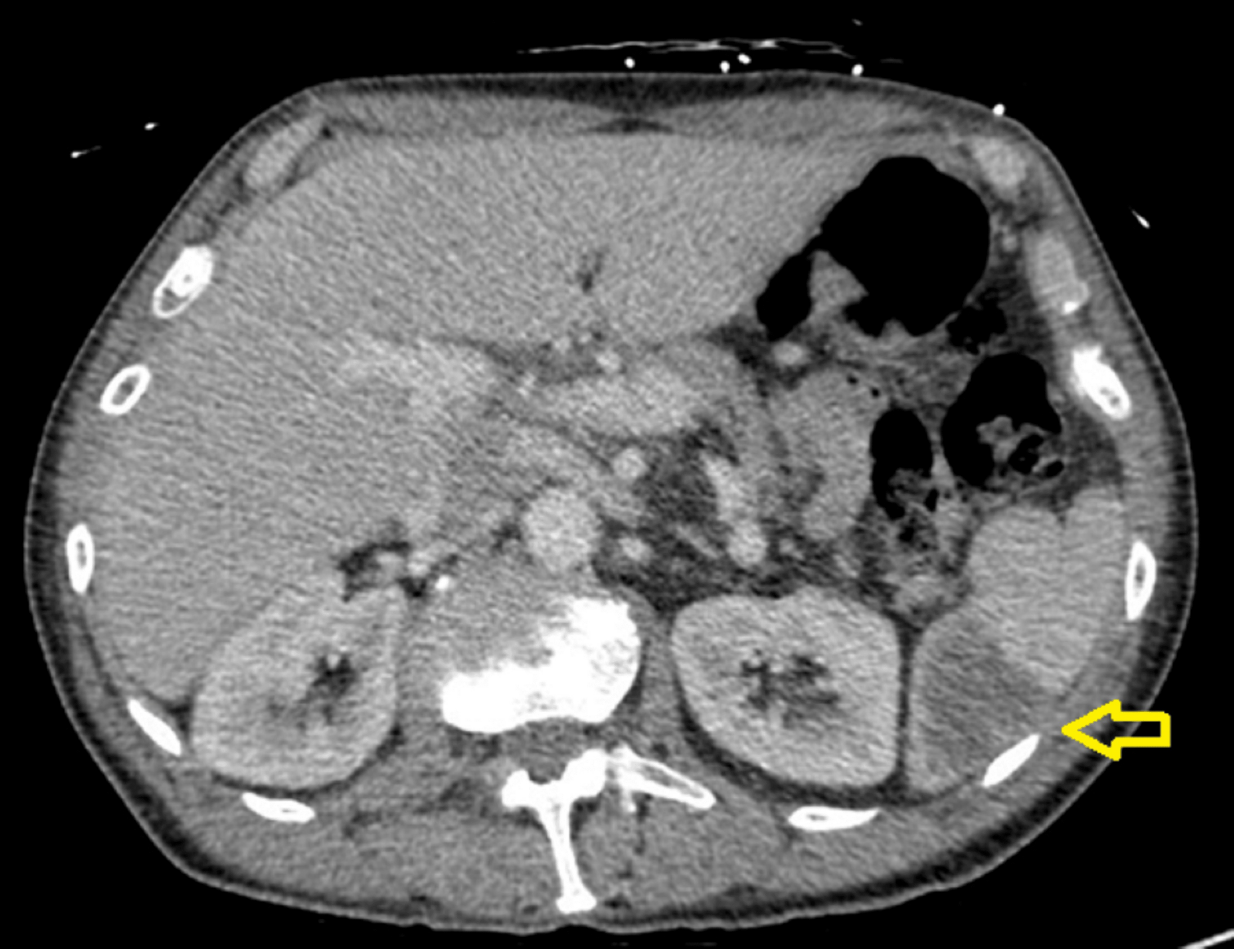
Computed tomography of the abdomen. Wedge-shaped low attenuation along the spleen, likely representing an infarct secondary to septic emboli from endocarditis, as indicated by the yellow arrow.
